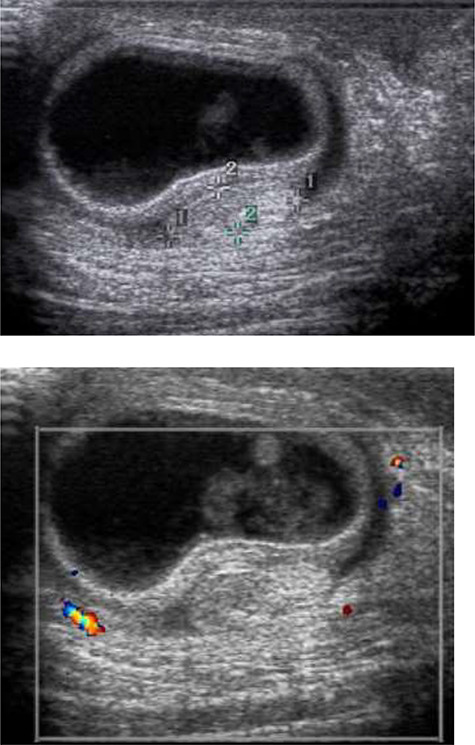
Ultrasound of the scrotum was performed assessing gray scale appearance and color Doppler flow. The right testis and inguinal region demonstrates a right inguinal hernia containing a loop of bowel within the right scrotum with echogenic bowel contents. A hyperechoic ovoid structured, favored to represent the right testicle, is seen measuring ~1.0 × 1.0 × 0.4 cm. There is no sonographic evidence of vascular flow with the right testicle.
